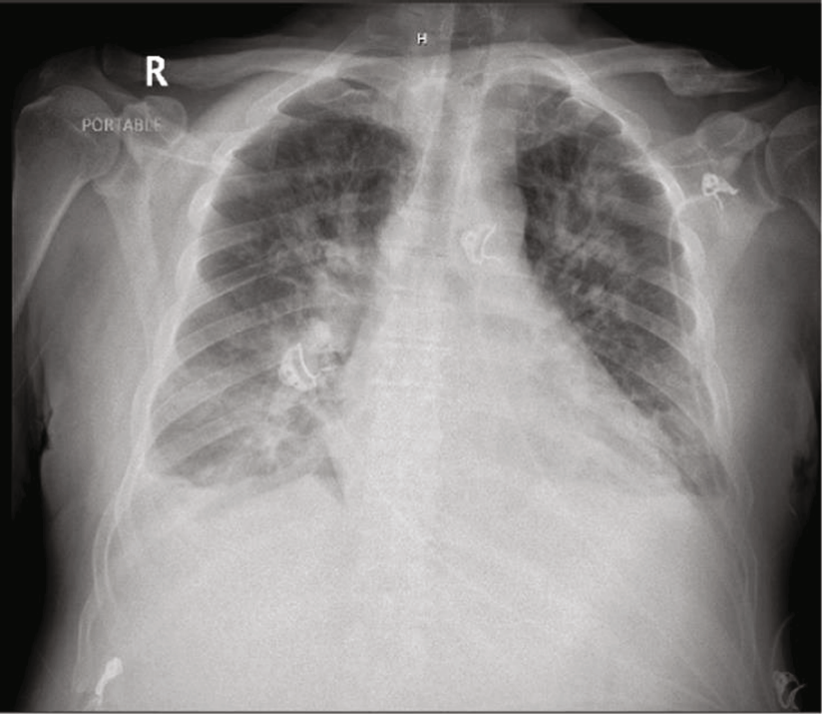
Chest X-ray: multifocal bilateral patchy ground-glass infiltrates with pleural effusions consistent with pulmonary edema.
